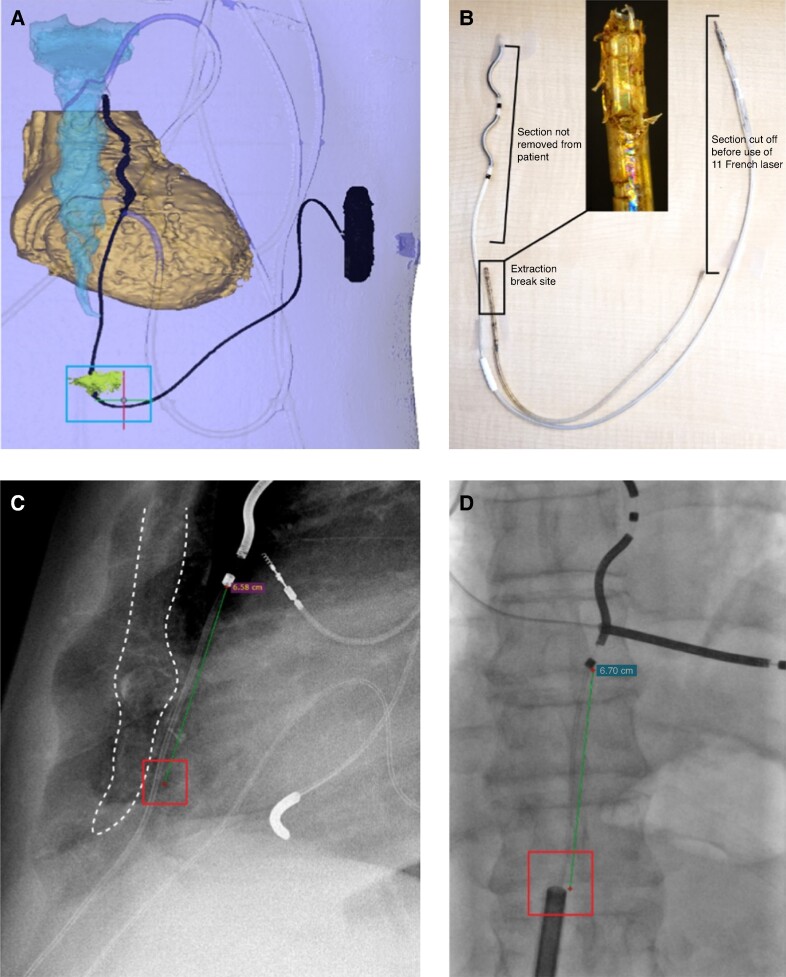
Images of chronic EV ICD lead that broke during extraction and was partially abandoned. 3D image of EV ICD lead placement before removal procedure; box at bottom of image demarcates the low midline subxiphoid incision site near the area with tough adhesions (*A*). Image of extracted portion of the lead next to a full-length lead for reference; close-up image is provided showing where extracted portion separated from abandoned portion of the lead (*B*). Lateral X-ray (*C*) and anteroposterior fluoroscopy (*D*) images showing sheath advancement into site of tough adhesions (boxes); sternum outlined with a dashed line in (*C*).
